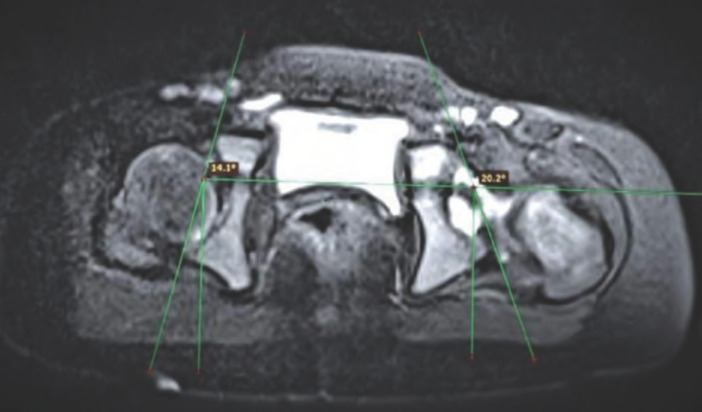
Acetabular anteversion (AA) angle: Axial T2-weighted fat-suppressed image of the hip in a patient with left sided DDH. The AA angle is 14.1° on the right side and increased (20.2°) on the left side.
